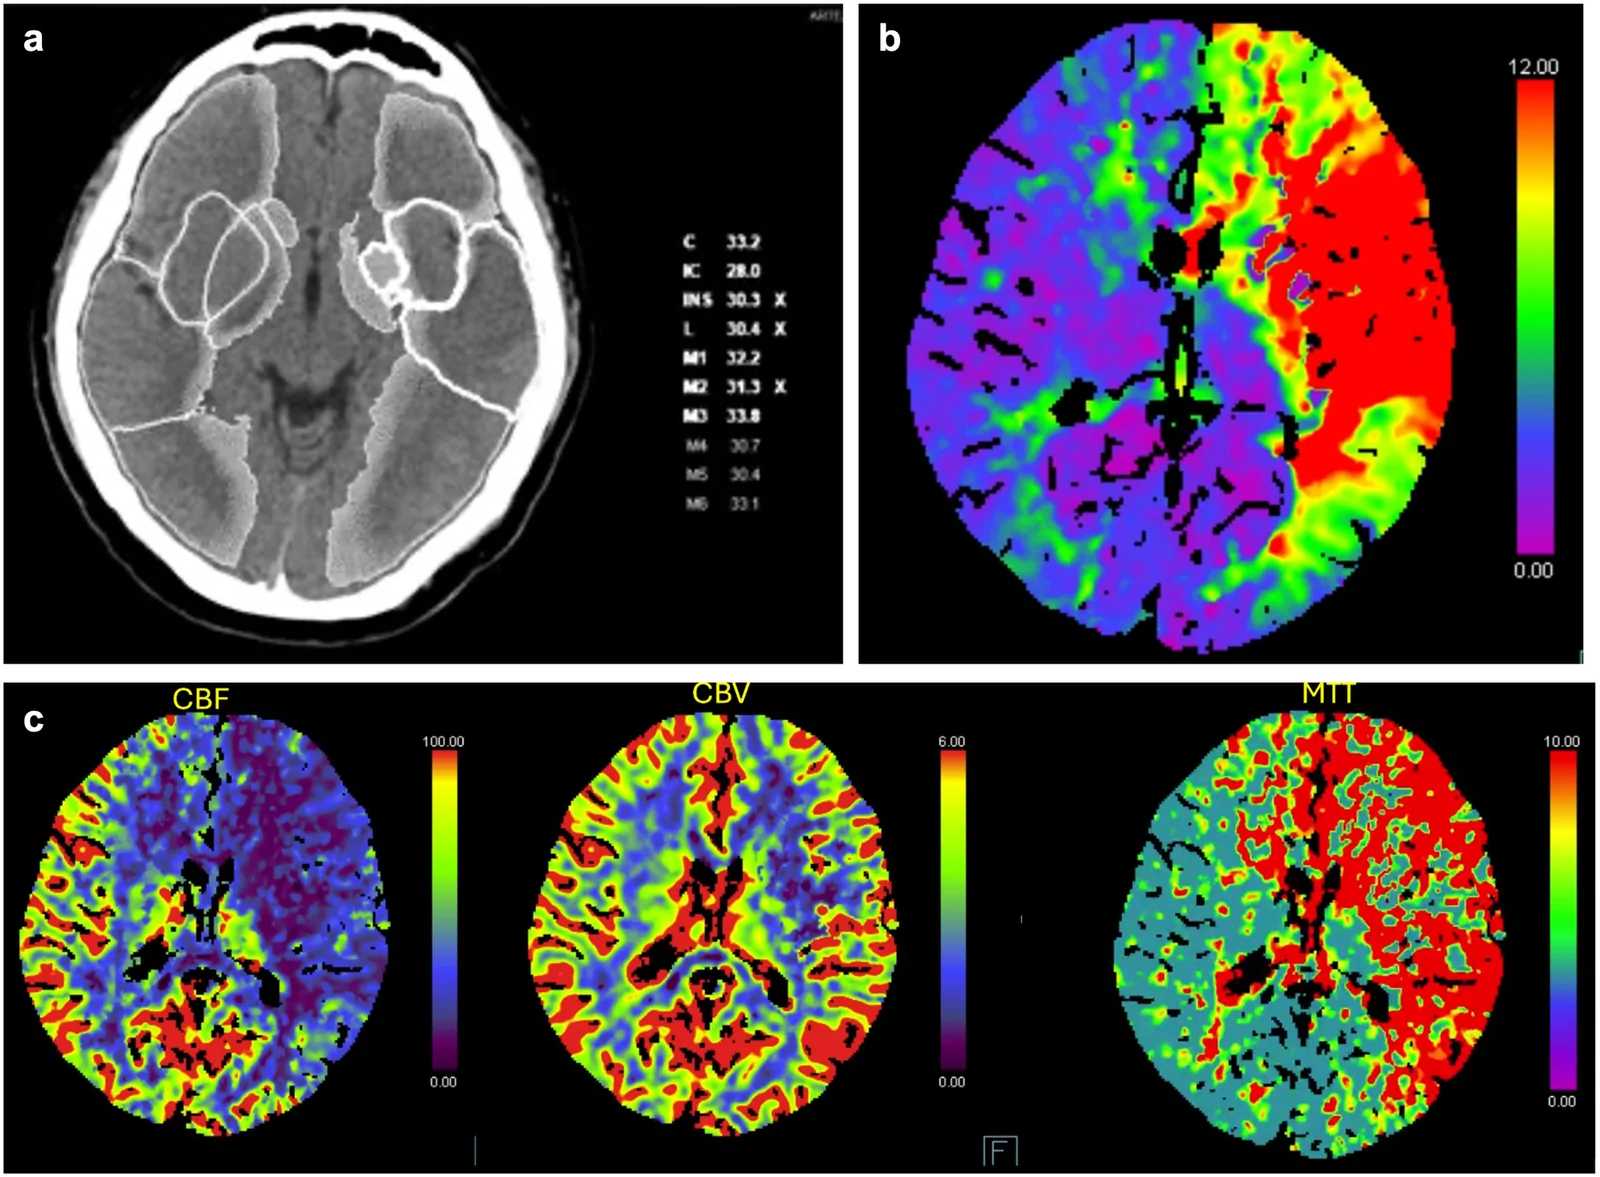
Initial non-enhanced CT and perfusion CT. (a) Early ischemic changes in the left MCA territory (ASPECTS 6: M2, M3, insula, lenticular). (b) Time-to-maximum. (c) Cerebral blood flow, cerebral blood volume and mean transit time, respectively. Penumbra: 385 mL. Core: 161 mL. Penumbra-Core mismatch: 2.39. The ischemic mechanism may have involved both reduced antegrade flow and thromboembolic phenomena related to the partially thrombosed aneurysm.
